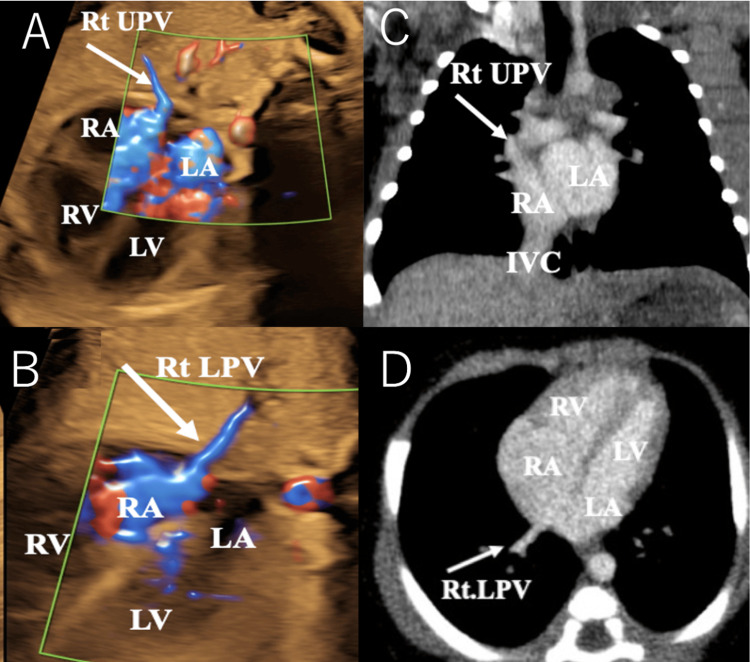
Fetal echocardiography and postnatal contrast-enhanced CT scan images A: Fetal echocardiography showing Rt UPV. B: Fetal echocardiography showing the Rt LPV. C: Postnatal contrast-enhanced CT confirmed that the Rt UPV, which normally drains into the LA, was abnormally connected to the RA. D: Postnatal contrast-enhanced CT confirmed that the Rt LPV, which normally drains into the LA, was abnormally connected to the RA CT: computed tomography; IVC: inferior vena cava; LA: left atrium; LV: left ventricle; RA: right atrium; Rt LPV: right lower pulmonary vein; Rt UPV: right upper pulmonary vein; RV: right ventricle
